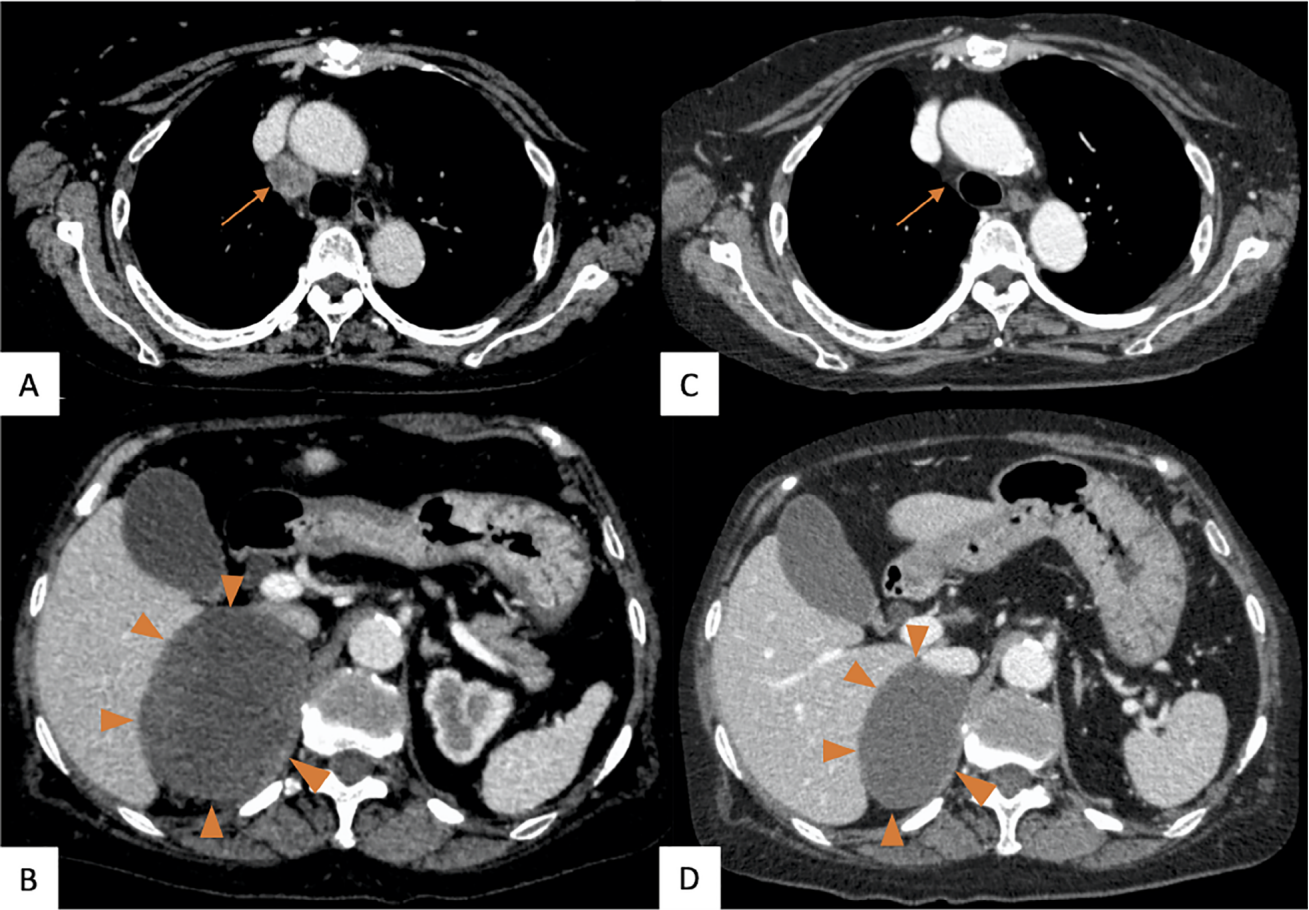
Computed tomography (CT) scans before and after chemoimmunotherapy. (A, B) Metastases to the mediastinal lymph node (arrow) and right adrenal (arrowheads) were observed three months after the surgery. (C) The shrinkage of the mediastinal lymph node (arrow) was confirmed after four courses of chemoimmunotherapy. (D) The right adrenal gland reduced in size from 81 × 65 to 61 × 43 mm (arrowheads).
